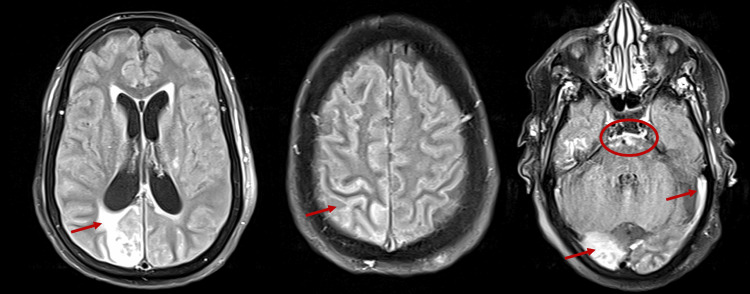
Magnetic resonance imaging (MRI) of the brain with fluid-attenuated inversion recovery sequence. The image a newly developed abnormal signal seen at the right high parietal region that exhibits a hyperintense signal, as well as a newly developed effacement of the pontine cisterns with abnormal signal intensity and abnormal signal of the cerebrospinal fluid seen at the extra-axial spaces.
